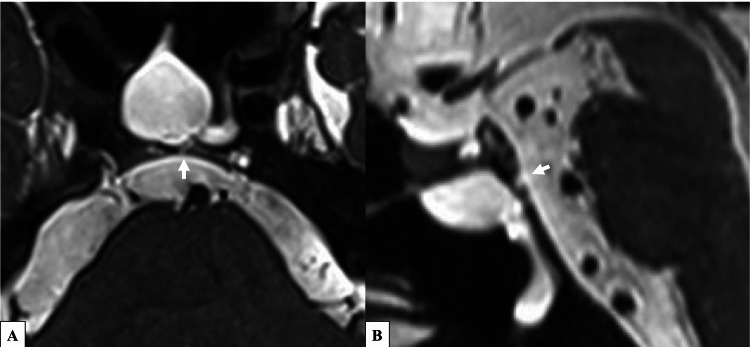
Preoperative magnetic resonance (MR) image of the area around the clivus. MR cisternography reveals a dural defect near the area of the bony defect identified by computed tomography. The white arrows indicate the dural defects.
